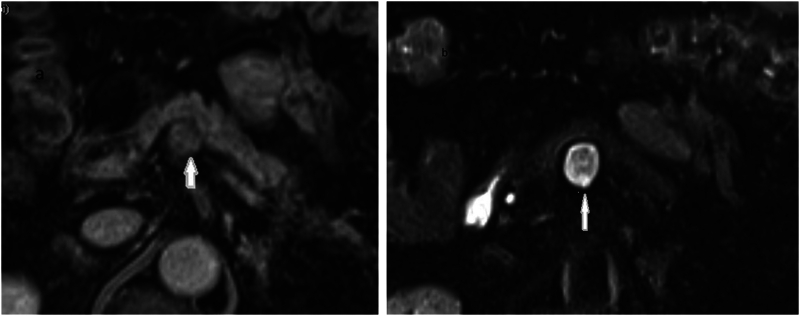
Pancreatic MRI. (a) Axial T1-weighted image (fat-suppressed, non-enhanced) showing a hypointense mass in the pancreatic body. (b) Axial T2-weighted image (fat-suppressed) depicting the lesion as heterogeneously hyperintense.
